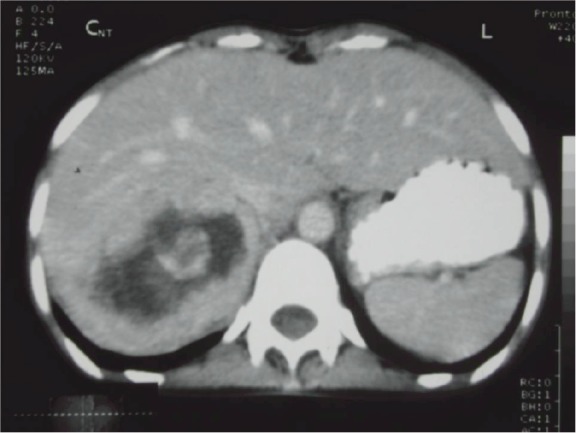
CT abdomen. Right adrenal mass with break down areas and calcifications.
